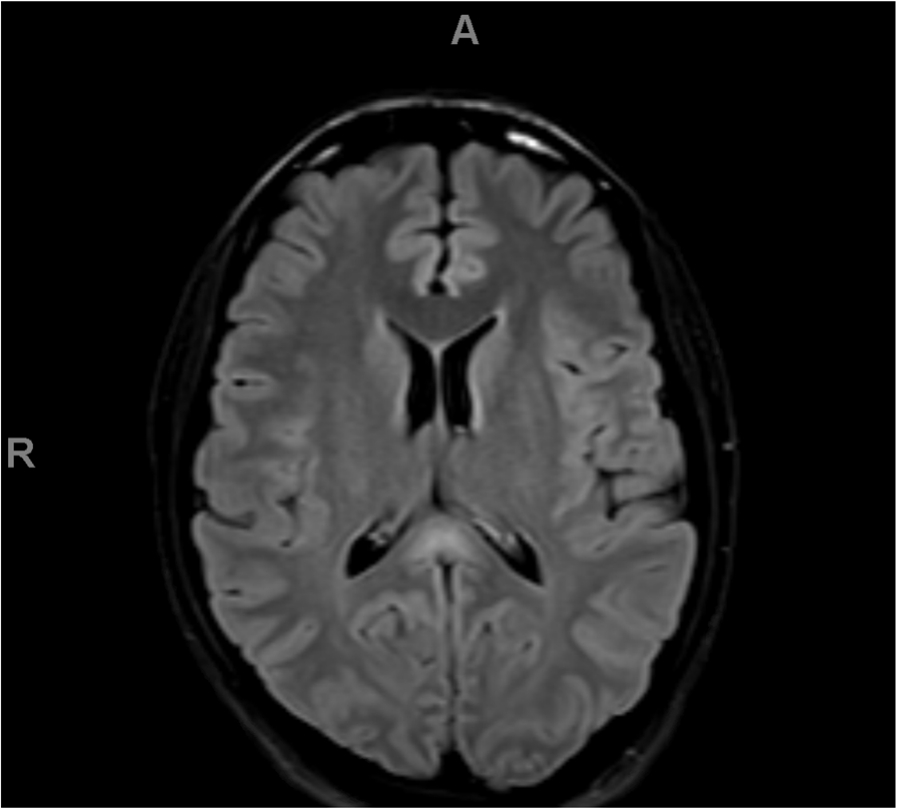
FLAIR axial images demonstrating high signal alterations in the splenium of the corpus callosum
